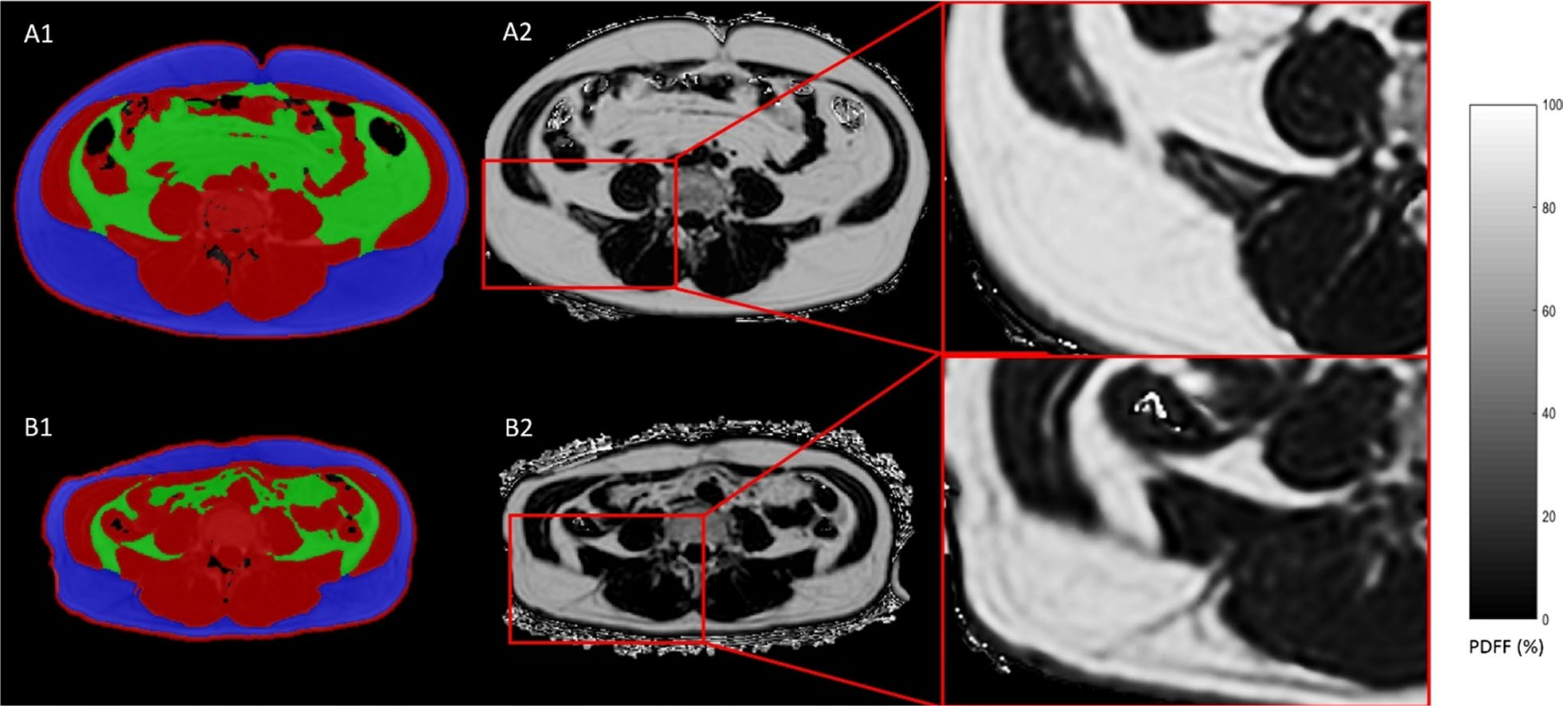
Adipose tissue changes in a patients with cancer cachexia: axial abdominal MRI images of a 54 year-old male subject with esophageal cancer at baseline (A) and follow-up after 8 months (B). A1, B1 Color-coded are VAT (green), SAT (blue) and non-adipose tissue (red). A2, B2 Corresponding PDFF maps showed a decrease in VAT from 83 to 72% and SAT from 87 to 76%. The BMI decreased from 30.2 to 24.1 kg/m2. *BMI* body mass index,* PDFF* proton density fat fraction,* VAT* visceral adipose tissue,* SAT* subcutaneous adipose tissue
